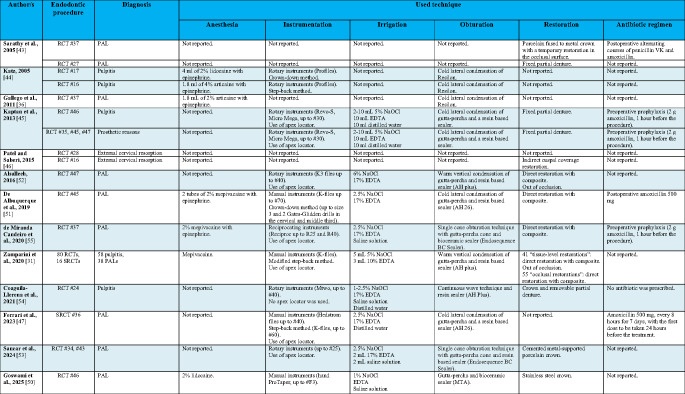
Tooth-related data from case reports, case series and prospective studies. Root Canal treatment (RCT), secondary root canal treatment (SRCT), periapical lesion (PAL)
